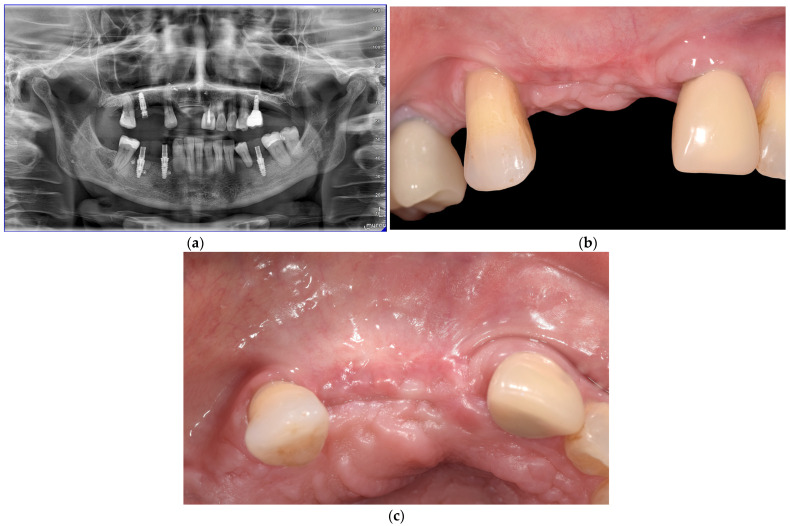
(a) Post-operative orthopantomography. (b) Frontal view of soft tissues healing after one month. (c) Occlusal view of soft tissues healing after one month.
